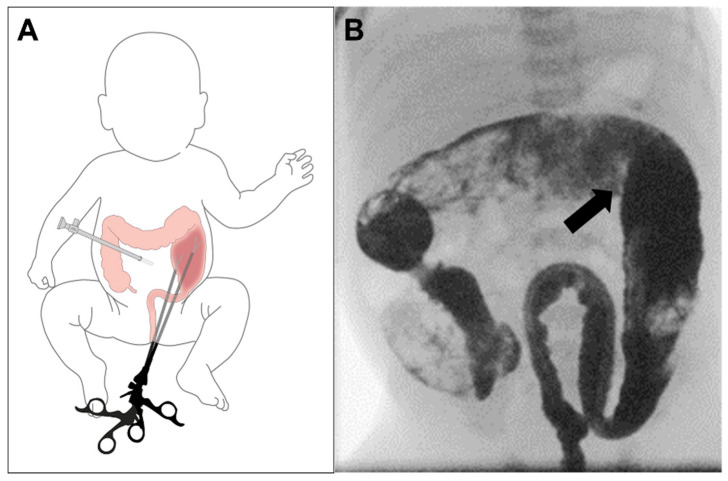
An umbilical camera port is placed, and full-thickness dissection is continued by transanal instrumentation entering the transition zone (A). The preoperative abdominal X-ray shows the transition zone (arrow) at the left colonic flexure (B).
